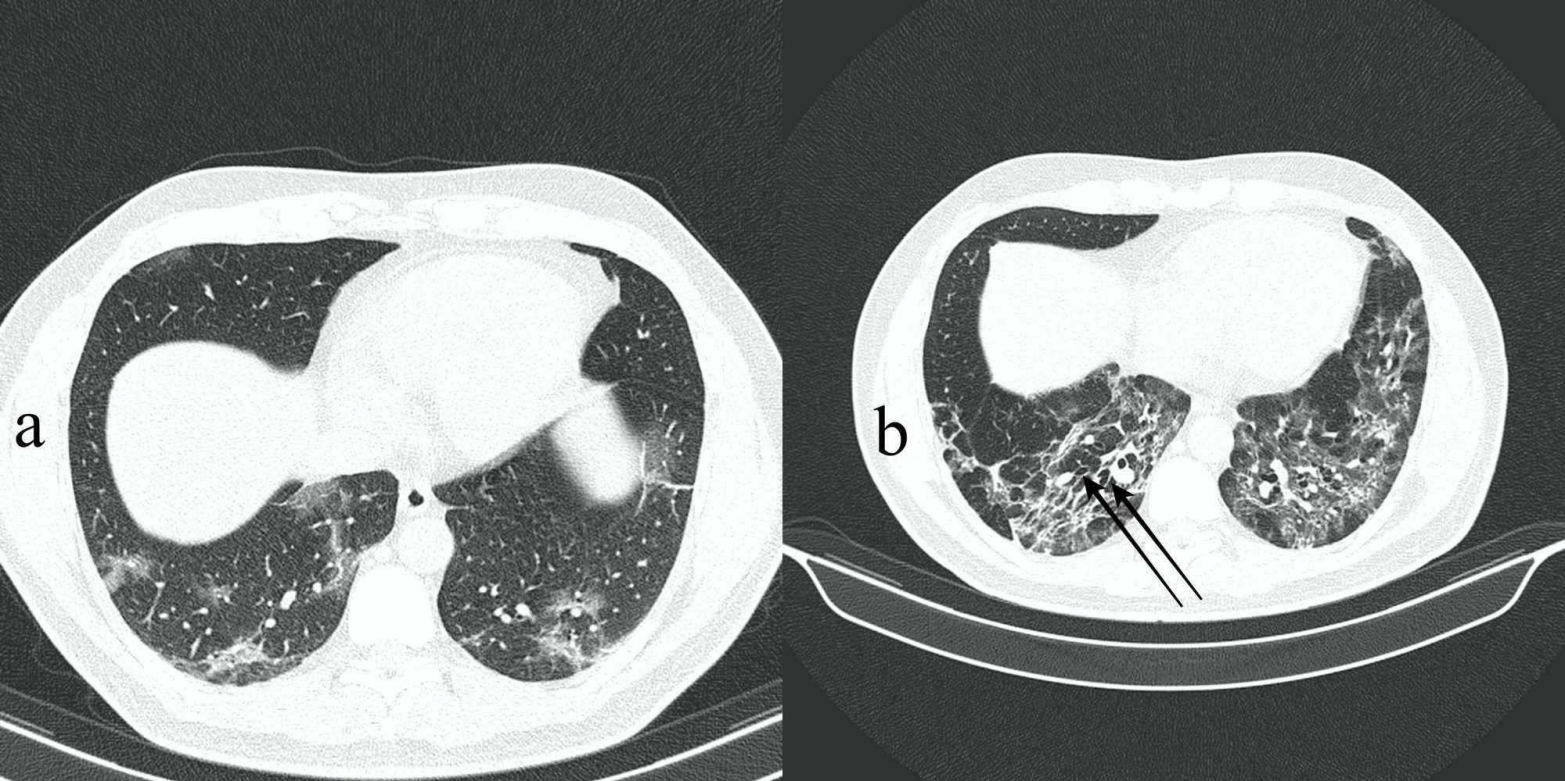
(a,b) Axial HRCT images showing new bronchiectasis and fibrotic changes as a sequel to severe respiratory disease (b, arrows). See initial baseline scan 35 days earlier without bronchiectasis for comparison (a). HRCT, high-resolution CT
